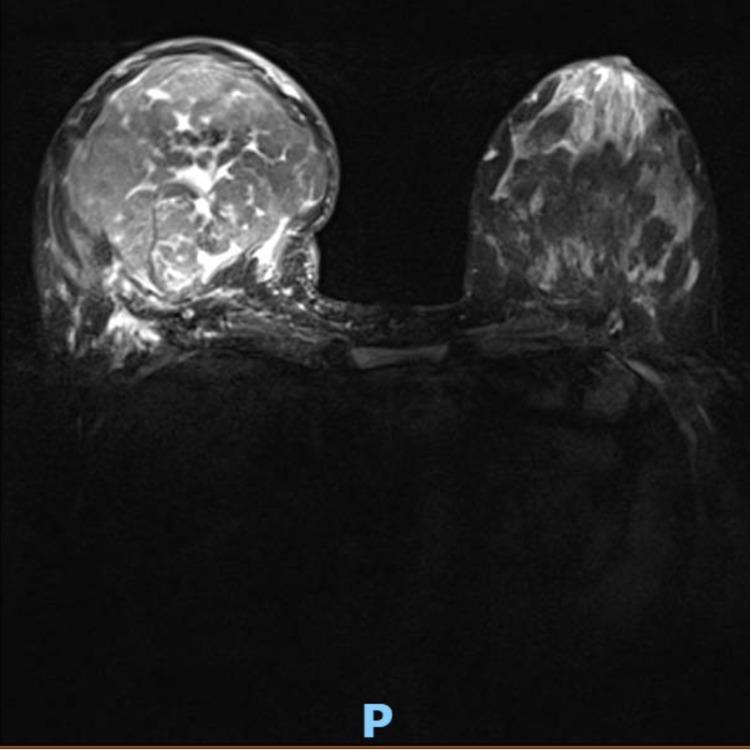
Bilateral breast MRI at 23 weeks showing 11.7 mm well-circumscribed hamartoma occupying the lower half of the breast with vivid heterogeneous enhancement and some central unenhanced dendritic-like areas.
